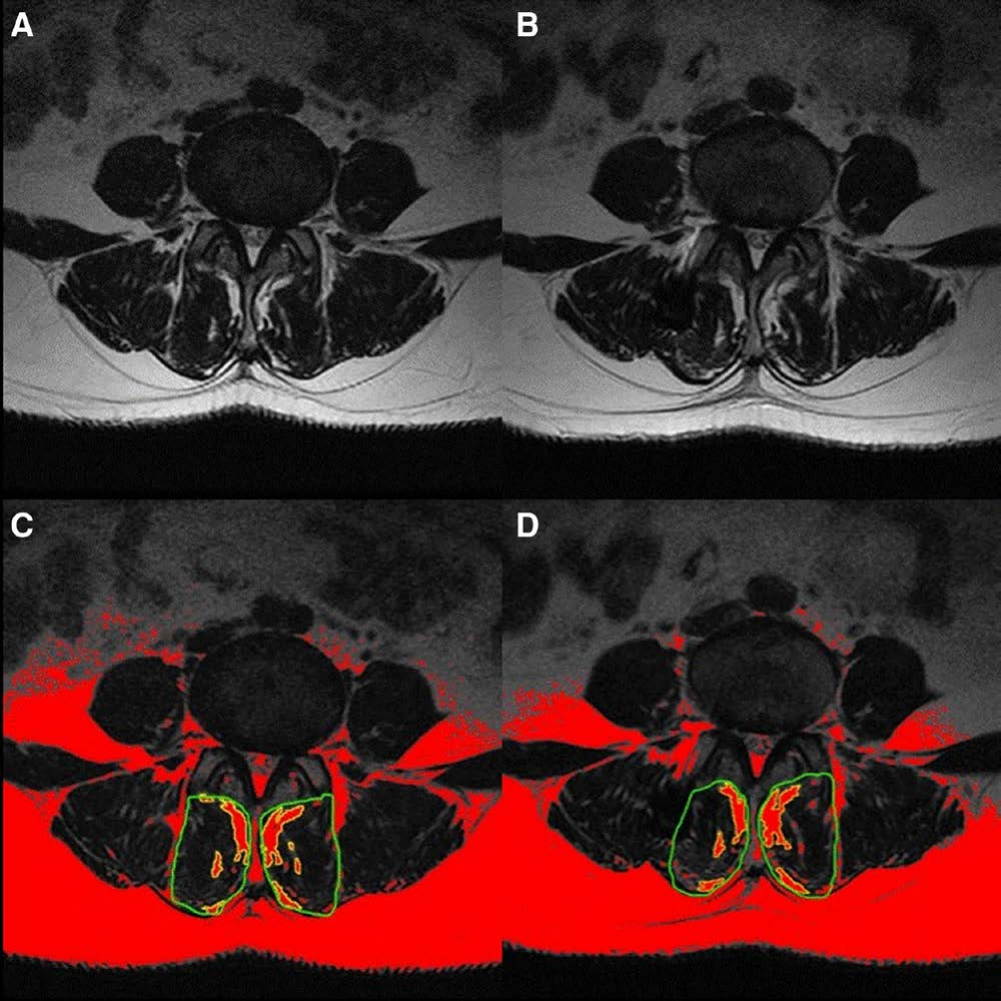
The Image J software was utilized to mark the multi-fissured muscle region (green border) in the selected MRI T2-weighted image, with red indicating adipose tissue and black-gray indicating muscle tissue. (A, C) MRI before surgery and images showing the measurement of fat infiltration rate. (B, D) MRI at 7-month follow-up after surgery and images showing the measurement of fat infiltration rate. MRI = magnetic resonance imaging.
